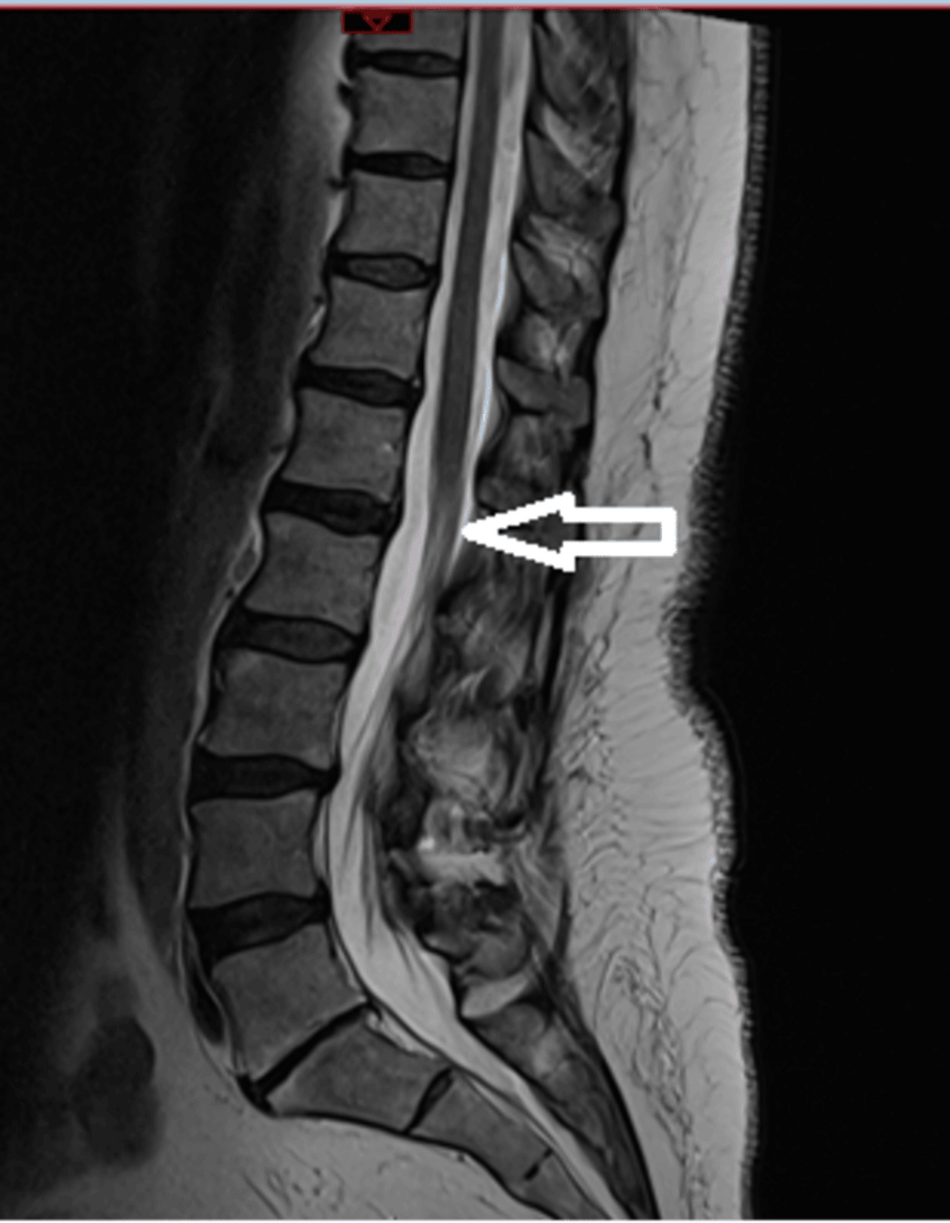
Sagittal T2‑weighted lumbar spine MRI demonstrating multilevel degenerative change without compressive cauda equina pathology No canal compromise or nerve‑root compression to account for cauda equina syndrome is identified.
